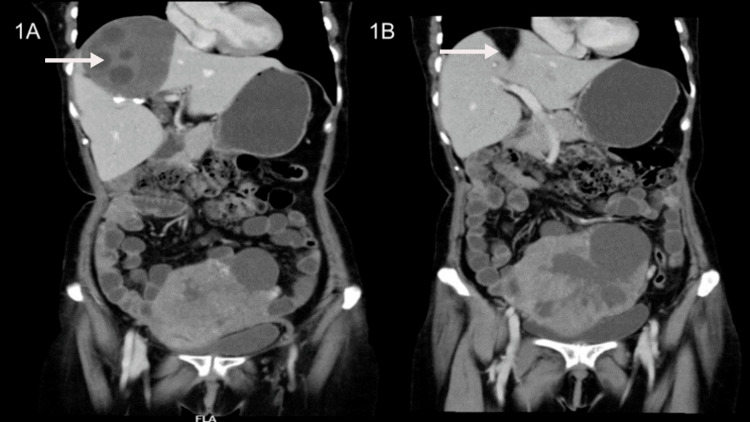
(A) Contrast-enhanced computed tomography of the abdomen and pelvis shows a complex solid-cystic enhancing lesion in the pelvis with an enhancing multicystic lesion in the liver. (B) (Post-chemotherapy) Contrast-enhanced computed tomography of the abdomen and pelvis shows a significant reduction in the size of the liver and ovarian lesion.
